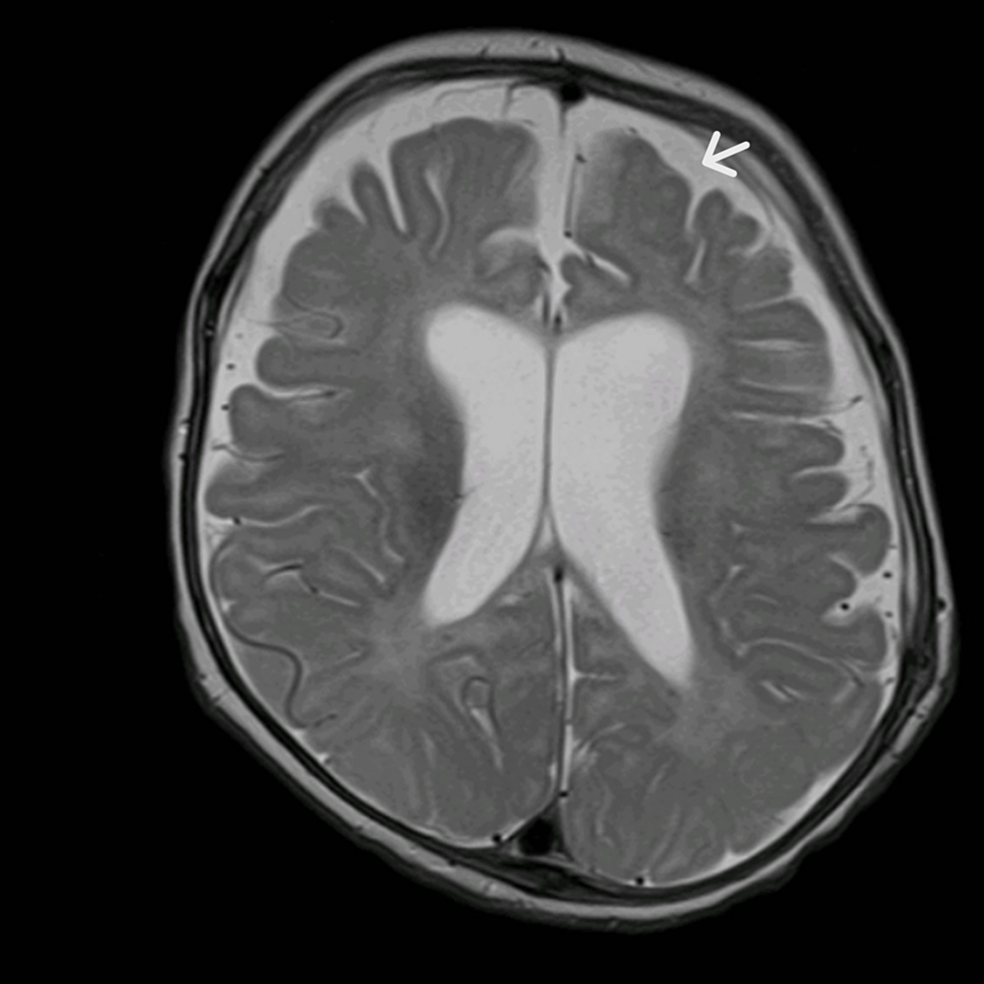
The final MRI of the brain performed prior to the discharge showed a decreased size of the previously observed left subdural fluid collection (white arrow)
